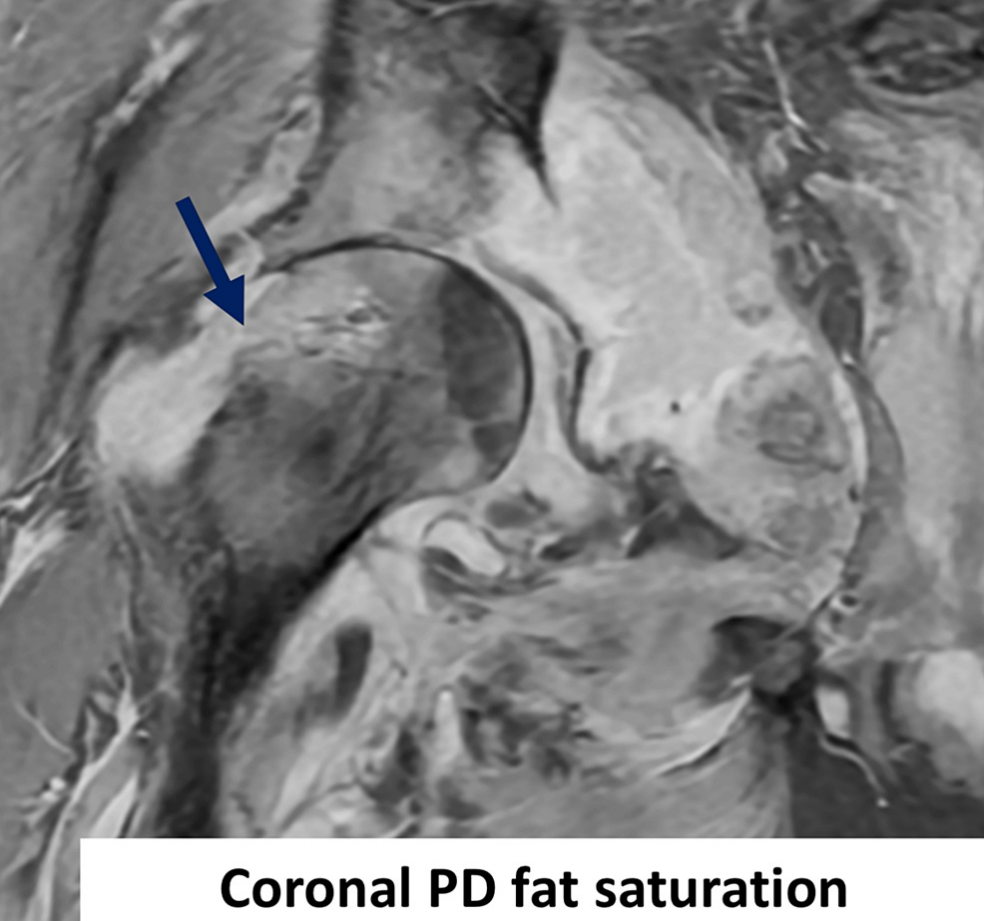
MRI of the right hip with coronal PD fat saturation. Interval development of erosive changes within the lateral aspect of the femoral head and neck (deep blue arrow). PD: proton density
